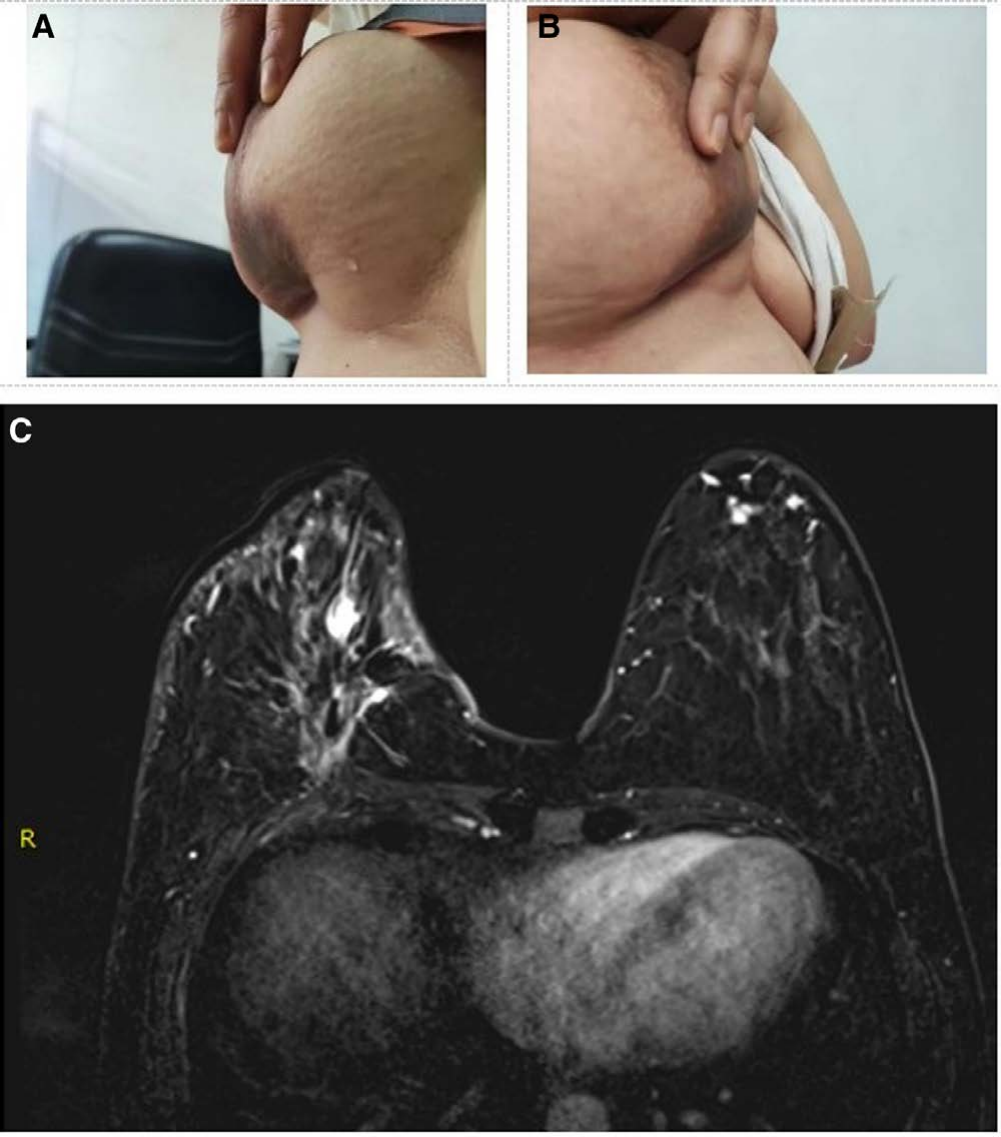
(A and B) Appearance of the breast after the comprehensive treatment. (C) Enhanced MRI of the breast after the comprehensive treatment. MRI = magnetic resonance imaging.
